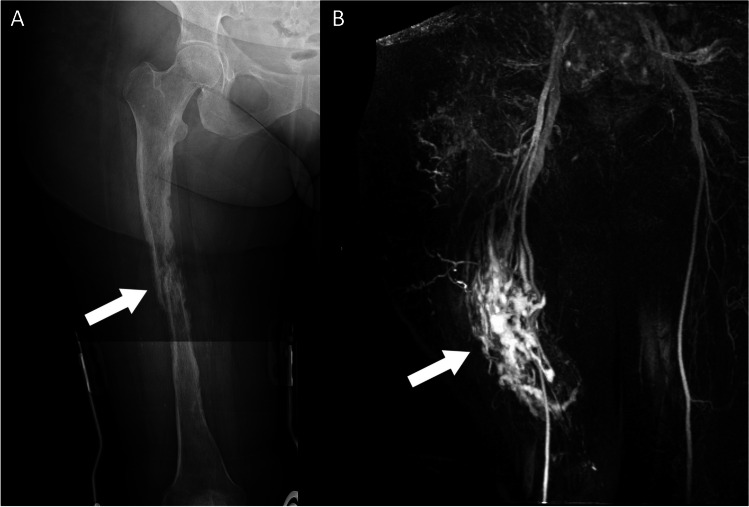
Axial (A and B) and sagittal (C and D) T1 and T2 fat-saturated MR images of the posterior thoracoabdominal wall in a patient with NF1 demonstrating hypertrophy of the skin (arrowhead) and subcutaneous fat, which is hyperintense on the T1W sequences (A and C), interspersed with a biopsy-proven diffuse plexiform neurofibroma that is hyperintense of the fluid sensitive sequences (D, white arrows). The whole area contains multiple convoluted flow voids (A and C, arrows)
